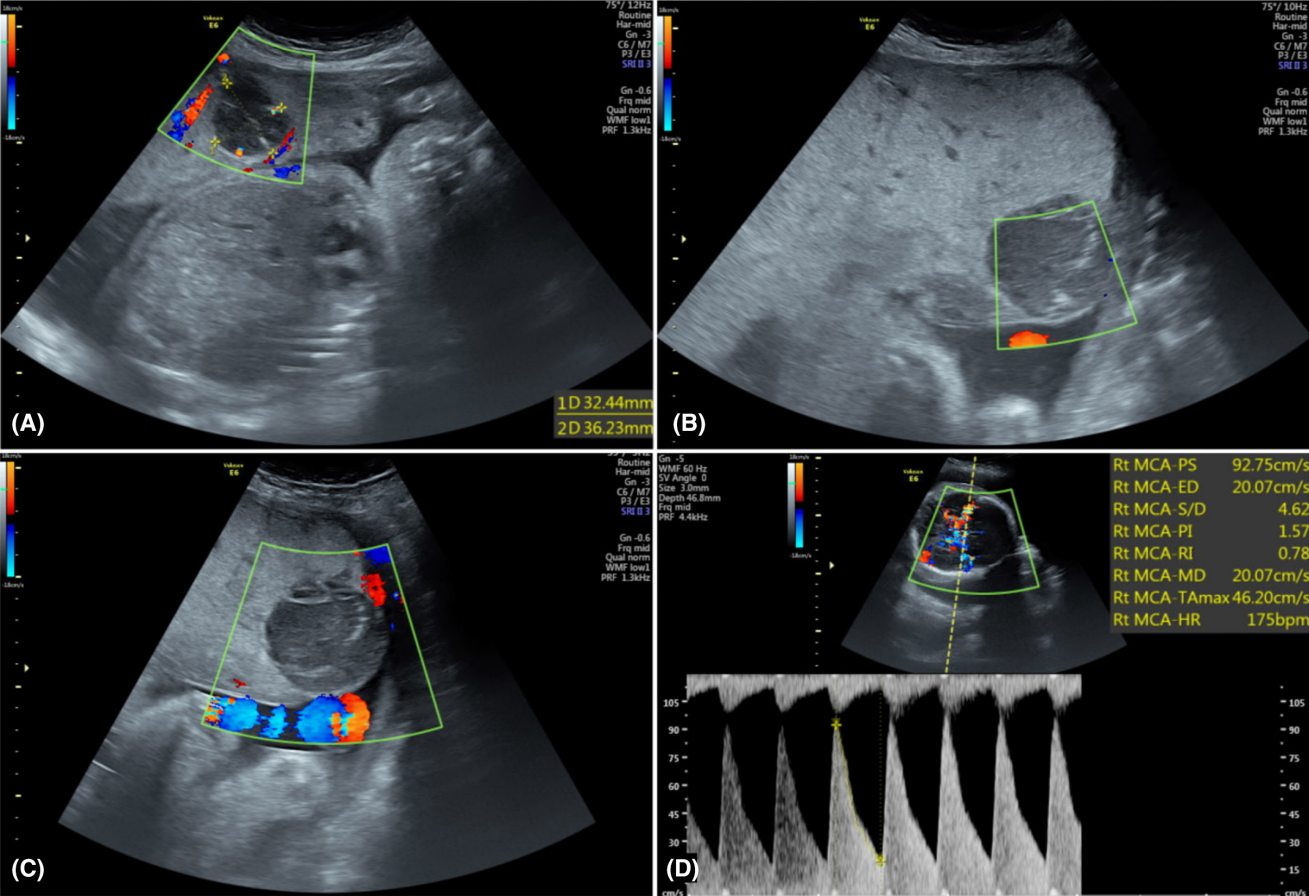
(A–C) The Doppler ultrasound of placental chorioangiomas showing the feeding vessels. (D) The MCA‐PSV measurement at 31 weeks of gestation corresponded to 2.14 multiples of the median (MoM).
